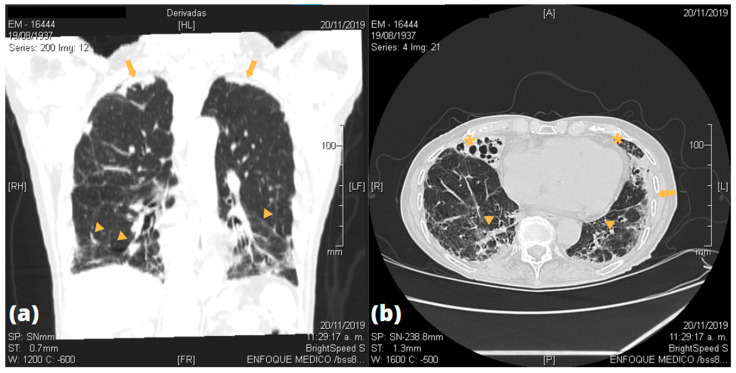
Images of an 85 years-old female patient with multiple HTLV-1 associated disorders (infective dermatitis, TSP/HAM, Sjögren’s syndrome). (a) Coronal CT scan shows peripheral images of ground-glass pattern in the lower lobes (arrowheads) and apical pleural thickening (arrows). (b) Transverse CT scan shows bilateral saccular bronchiectasis in the lower lobes (asterisks), thickening of bronchovascular bundles, and presence of ground-glass pattern (arrowheads) with pleural thickening (arrow).
